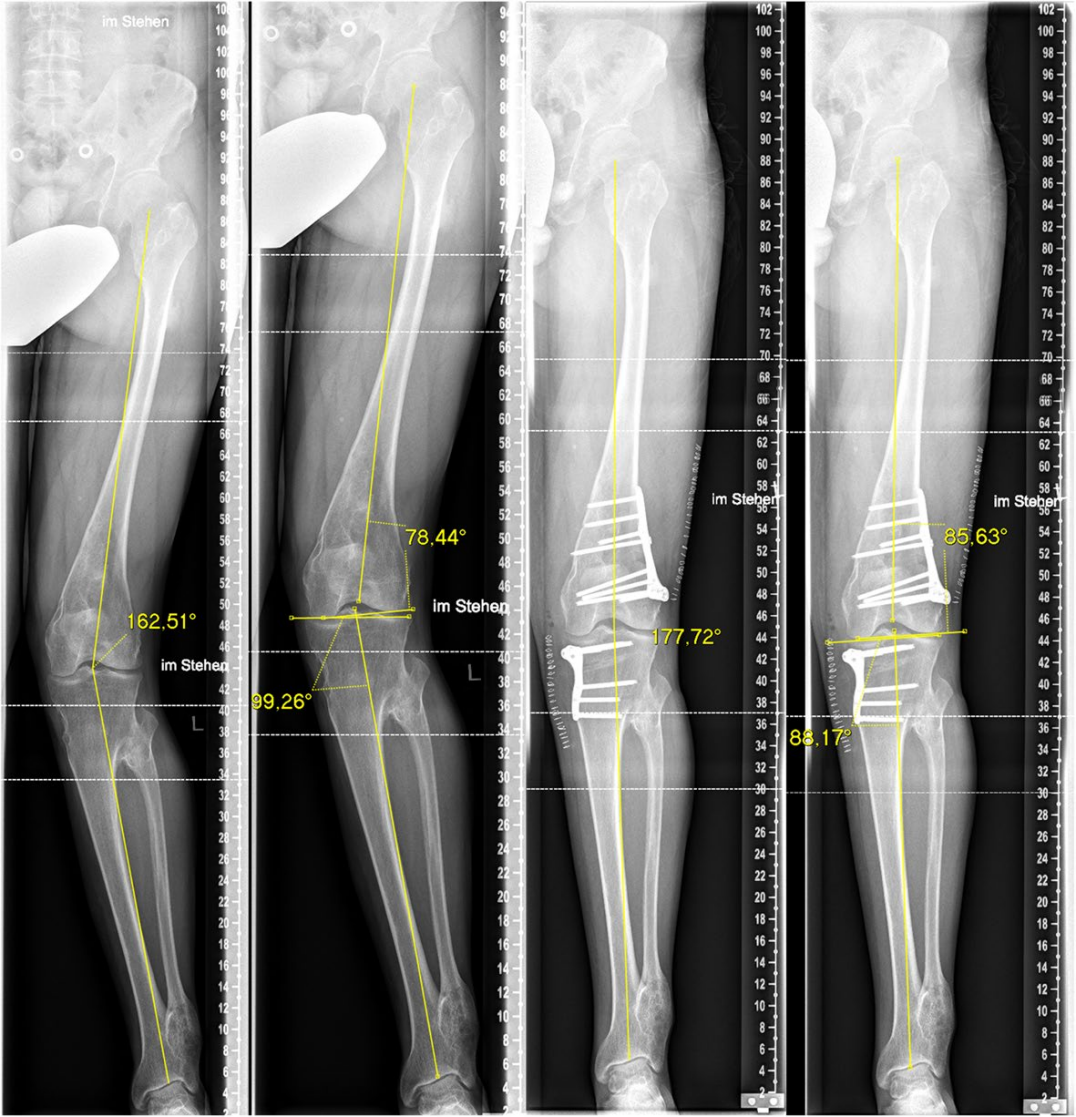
The accuracy of the procedure was defined as the delta (∂) between planned correction and postoperative alignment measured on full weight bearing long leg radiographs (∂ HKA A + C, ∂ MPTA B + D, ∂ LDFA B + D)
